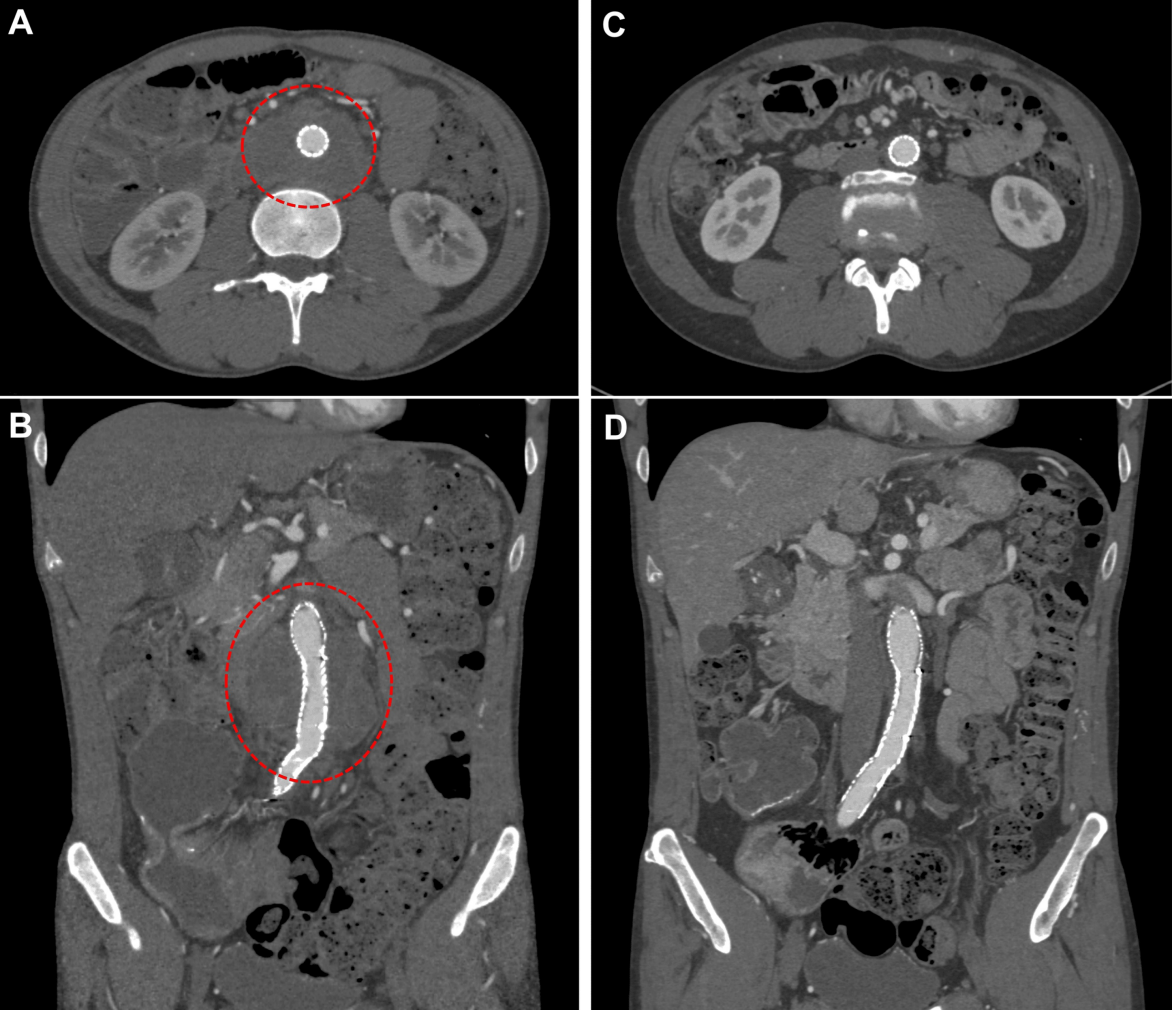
Postoperative computed tomography images Images obtained (A, B) at one week and (C, D) at five years after surgery, showing shrinkage of the aneurysm. The circles indicate the aneurysmal sac.
